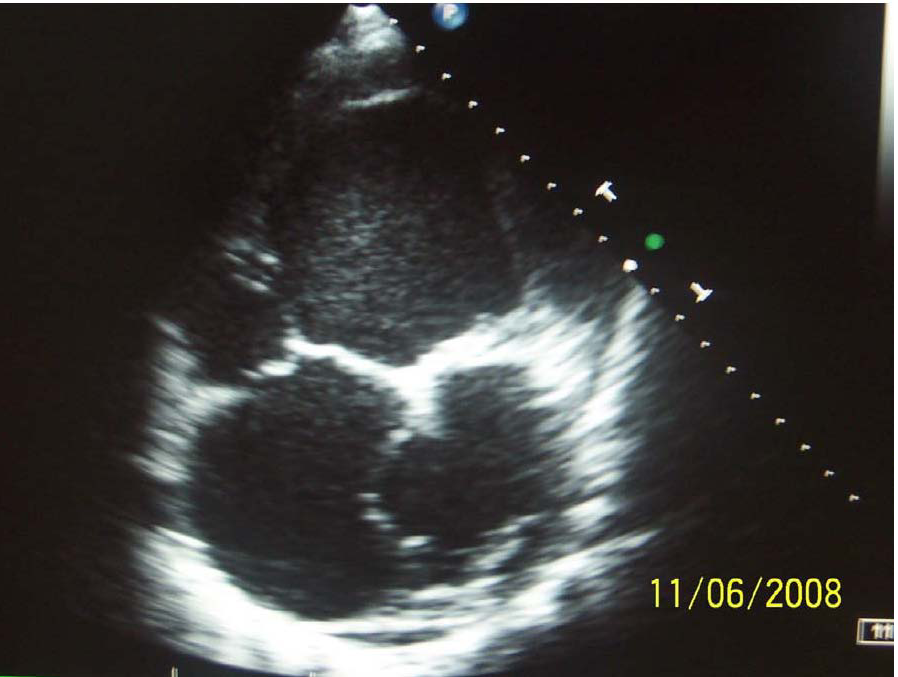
Apical four chamber view, single ventricle interatrial aneurysm.
